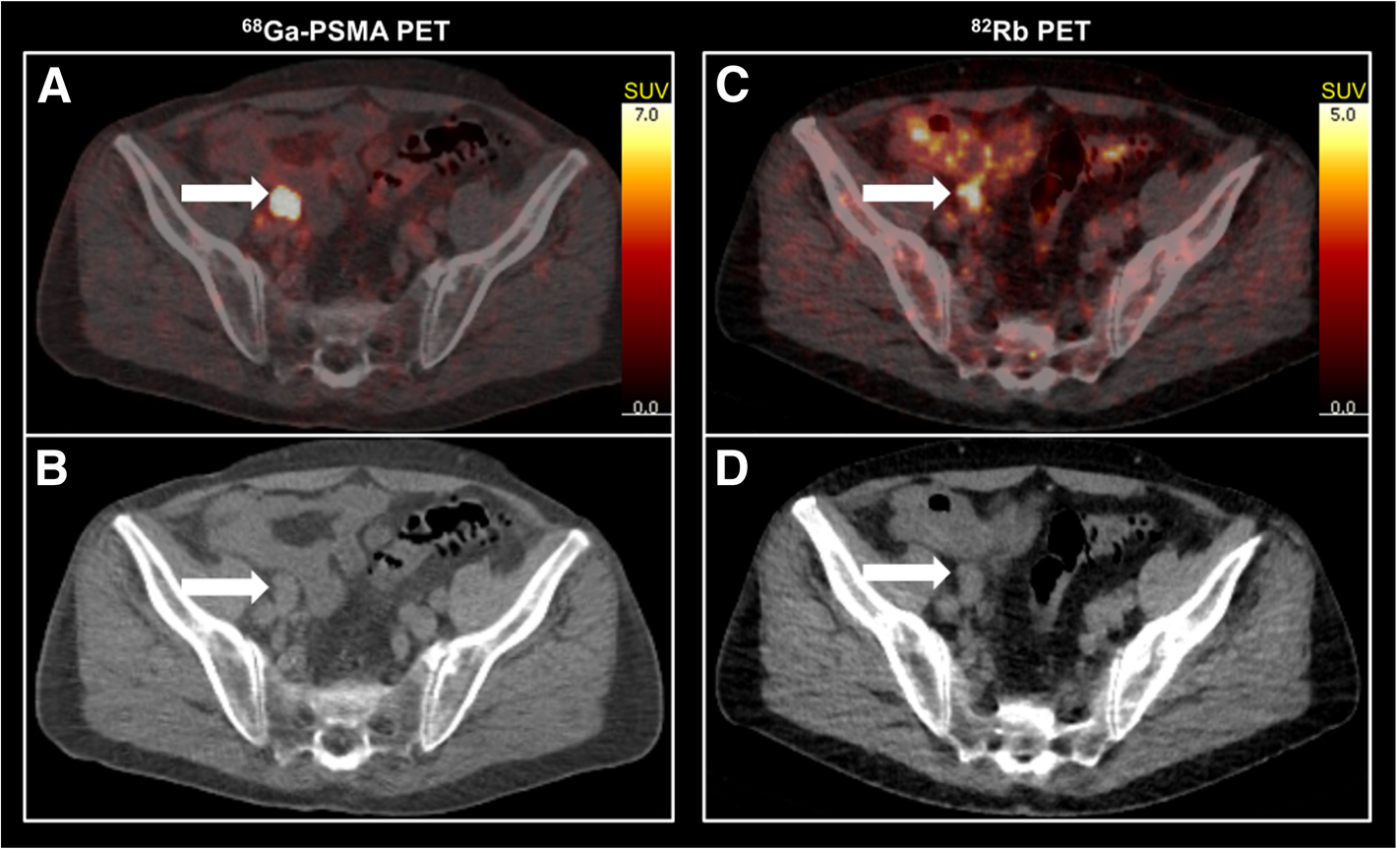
Images of a pelvic lymph node metastasis (patient 2). To the left, ^68^Ga-prostate-specific membrane antigen (PSMA) positron emission tomography (PET)/computed tomography (CT)-fused image (a) (SUVmax 43.0) and corresponding low-dose CT (b). To the right, ^82^Rb PET/CT fused image (c) (SUVmax 6.5) and corresponding low-dose CT (d)
